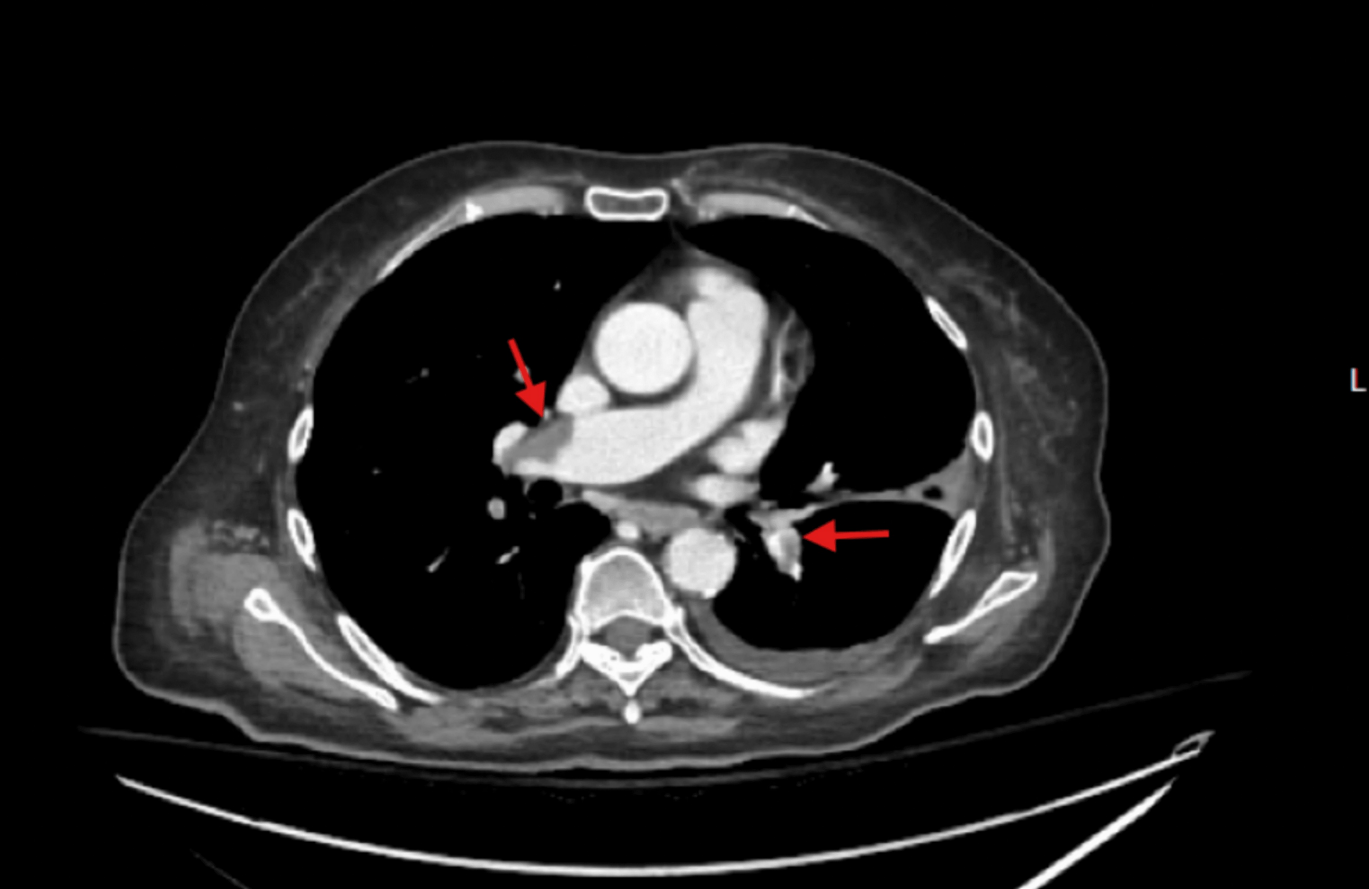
CT image showing bilateral pulmonary emboli (arrows). This patient had systemic thrombi that embolized to the pulmonary system.
